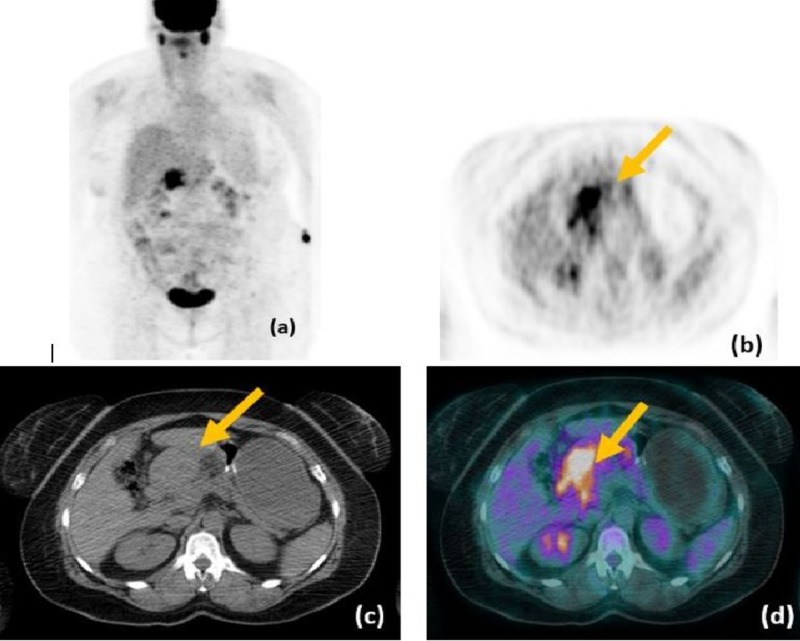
PET/CT scans A-D: Images from the same patient demonstrate marked hypermetabolism within the gastric pylorus mass. PET: positron emission tomography; CT: computed tomography
